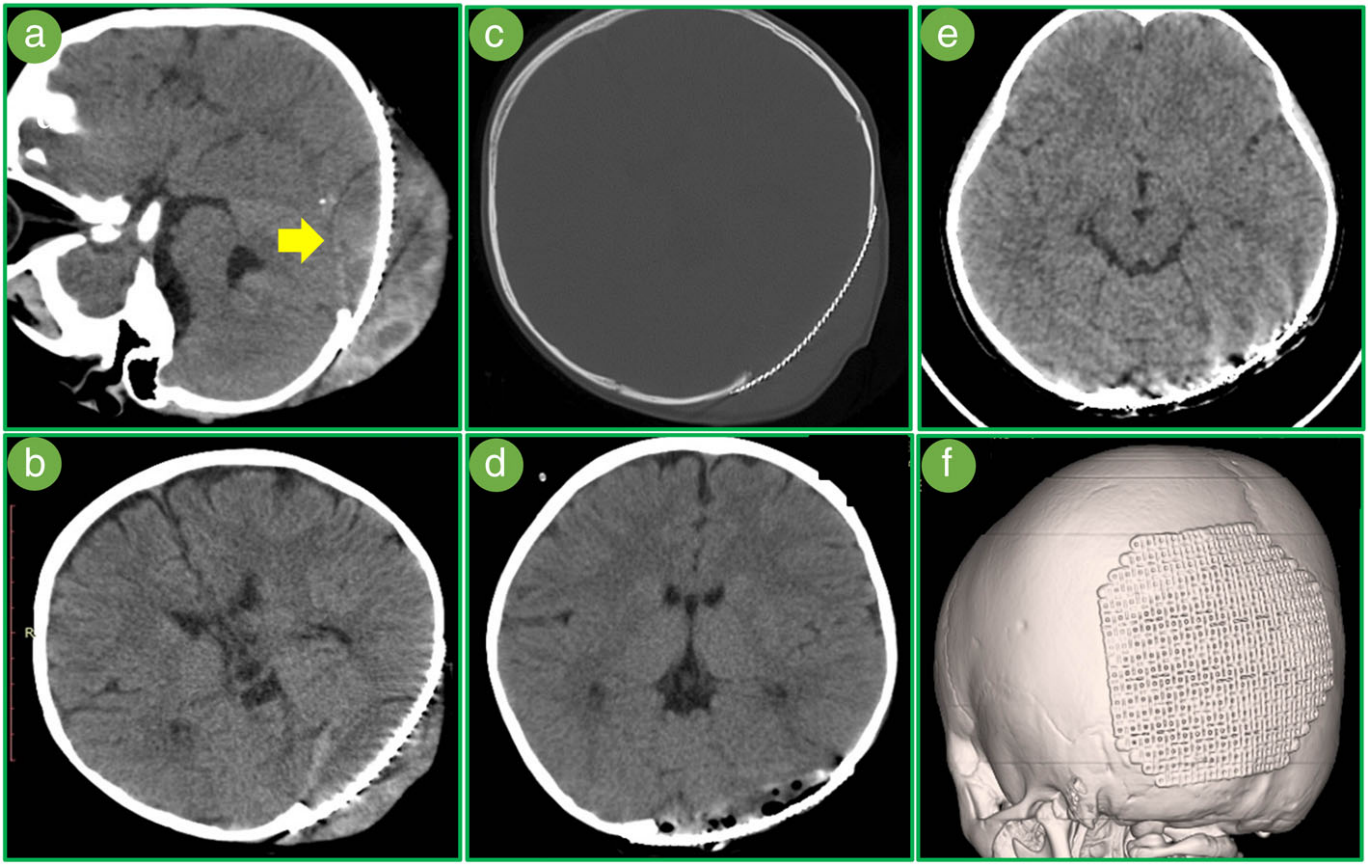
a After puncturing, a compression caused by epidural hematoma was found on CT (yellow arrow). b Postoperative CT found a hematoma (24 h after surgery). c The mesh was fixed well. d Four days after surgery, hematoma was obviously absorbed. e, f Three months of surgery, the mesh was well fixed, no bleeding and subcutaneous hydrops
